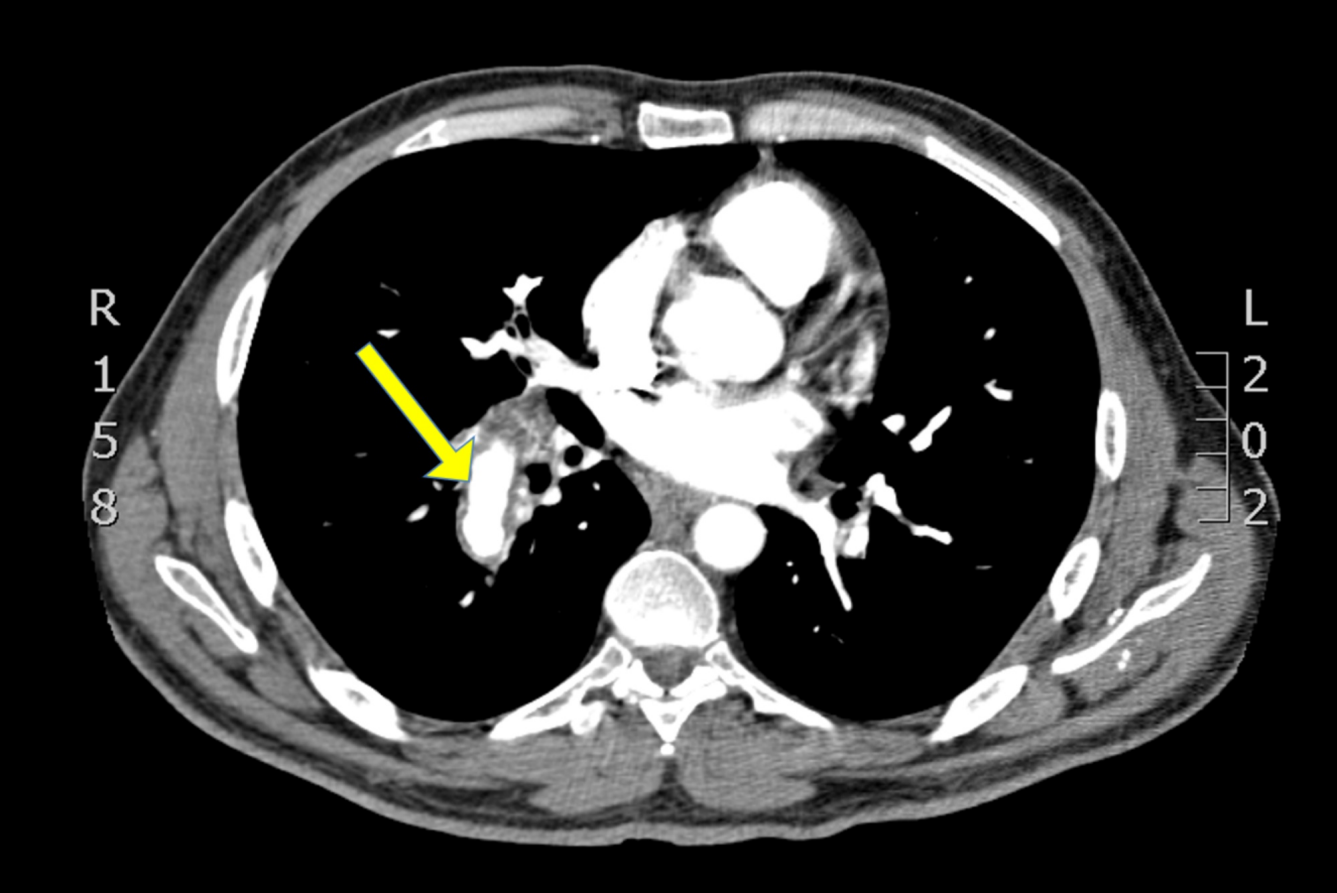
Chest CT after fourth cycle of chemotherapy showing dilated right pulmonary artery with wall thickening CT, computed tomography
